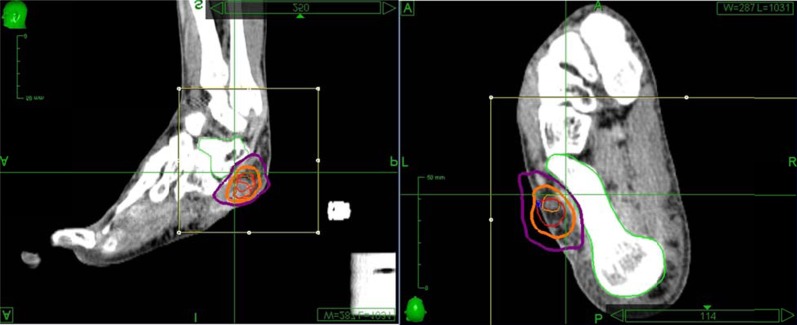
The CyberKnife treatment plan utilizing-fused CT and MRI scans of the left foot. The gross tumor volume was outlined on both scans and prescribed a dose of 3000 cGy. Thin orange is the CT-GTV, which was treated to a minimum of 3122 cGy and a maximum of 4139 cGy. Thin red represents the MRI-GTV, which was treated to a minimum of 3289 cGy and a maximum of 4225 cGy. Each GTV received 100% of the prescribed dose. The two GTVs were fused with an extension of 2 mm to create the CTV (thick orange) and a margin of 5 mm was added (thick purple) was added to create the PTV. The talus was outlined and kept to a maximum of 2260 cGy.
